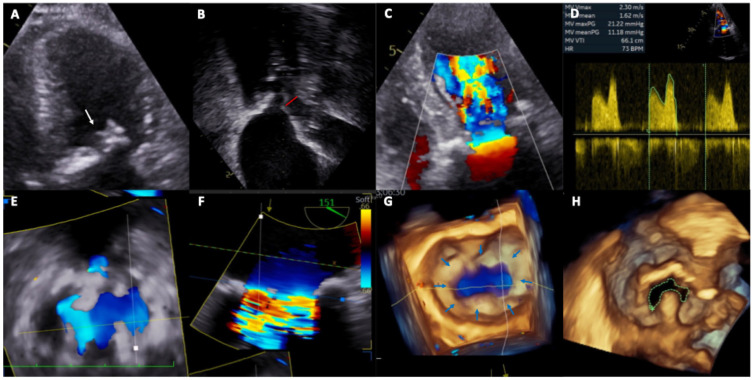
Pre-procedural echocardiography assessment. (A) Transthoracic echocardiography showed mitral leaflets and annular calcification (white arrow). (B) Transthoracic echocardiography left ventricle outflow tract assessment (red line). (C) Transthoracic echocardiography color aliasing showed mitral stenosis. (D) Transthoracic echocardiography Pulse wave Doppler showed severe mitral stenosis. (E) Transesophageal echocardiography showed severe anulus mitral calcification. (F) Transesophageal echocardiography color aliasing showed mitral stenosis. (G) 3D Transesophageal echocardiography showed circumferential mitral annular calcification (blue arrows). (H) 3D Transesophageal echocardiography showed reduced planimetric mitral valve area.
